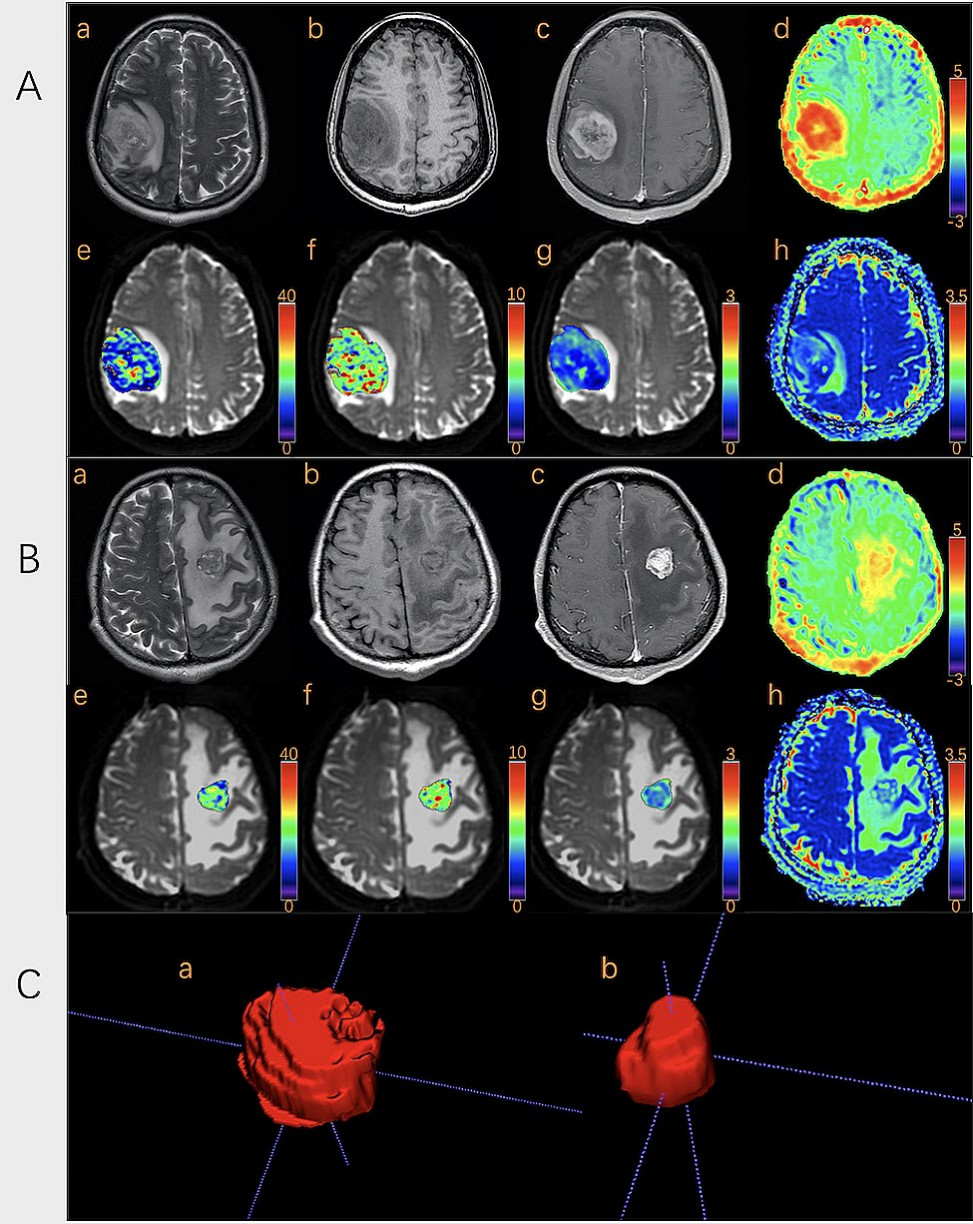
Representative images of patients with glioma or metastases. (Row A & Row B): a: T2W, b: T1W, c: contrast-enhanced T1W, d: MTRasym@3.5ppm, e: Dfast(×10^− 3^mm^2^/s), f: Dslow(×10^− 3^mm^2^/s), g: frac (%), h: ADC(×10^− 3^mm^2^/s). Row C: 3D reconstruction of tumor ROI of glioma patient (a) and SBM patient (b). Row A: A 63-year-old woman pathologically confirmed with glioblastoma. MRI showed an irregular lesion with unclear margin in the right frontal lobe, presenting as a diffuse enhanced lesion with hyper-intensity on T2W and hypo-intensity on T1W accompanied by peritumoral edema. Histogram features of the enhanced tumor parenchyma are as follows: ADC_kurtosis_:4.84. (MTRasym@3.5ppm)_10_: 1.5, frac_10_:7.38, frac_90_:18.79, frac_mean_:12.85, frac_entropy_:3.26, frac_kurtosis_:4.92, frac_skewness_:0.90. Row B: A 34-year-old woman was pathologically confirmed with adenocarcinoma; a primary lesion was found in her left lung. MRI showed a regular lesion with a clear margin in the left frontal lobe, presenting as a diffuse-enhanced lesion with iso-intensity on T2W and T1W accompanied by peritumoral edema. Histogram features of the enhanced tumor parenchyma are as follows: ADC_kurtosis_:2.74. (MTRasym@3.5ppm)_10_: 1.9, frac_10_:12.62, frac_90_:21.94, frac_mean_:17.21, frac_entropy_:3.95, frac_kurtosis_:3.22, frac_skewness_:0.13
